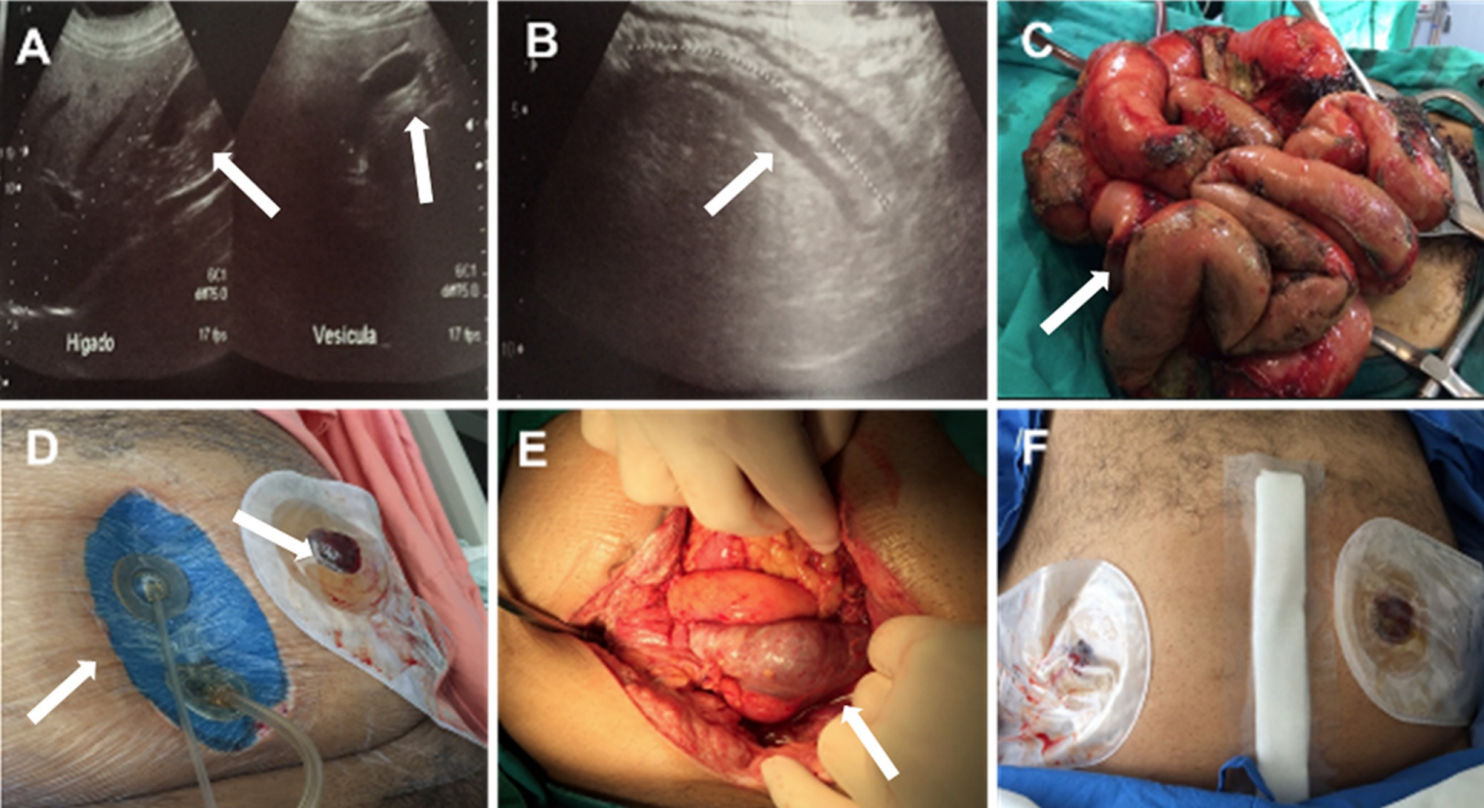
Perforated diverticulitis and diffuse peritonitis A. Abdominal ultrasound showing cholecystolithiasis (arrows); B. Abdominal ultrasound showing acute appendicitis (arrow); C. Active diffuse suppurative peritonitis; D. Abdominal installation and temporary colostomy; E. Open abdomen 72 hours after abdominal instillation (arrow); F. Closed abdomen
